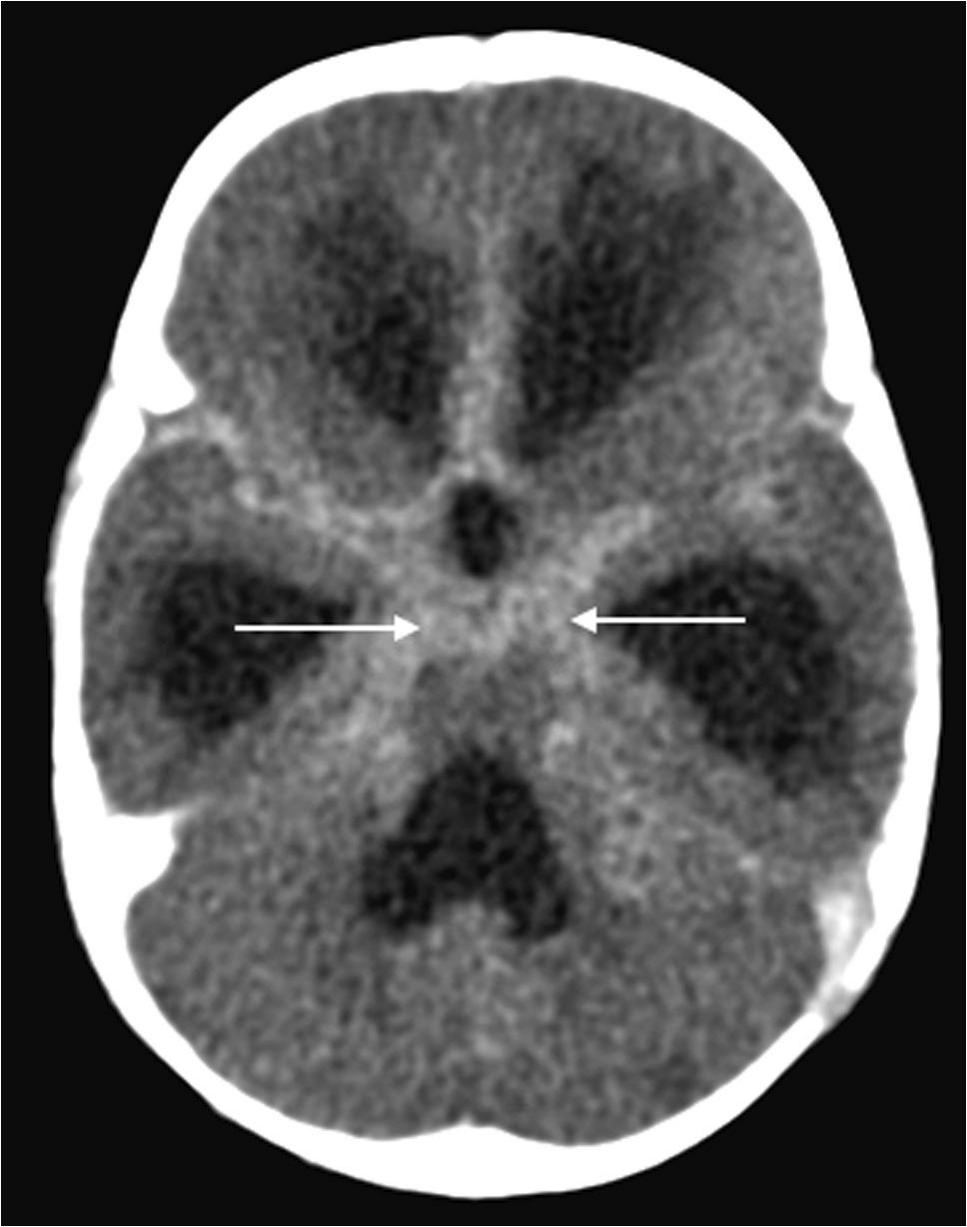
Axial post-contrast  computed tomography image of the brain in a 30-month-old boy with tuberculosis (same patient as Fig. 12) shows diffuse basal meningeal enhancement filling the cisterns (*arrows*) and accompanying hydrocephalus
